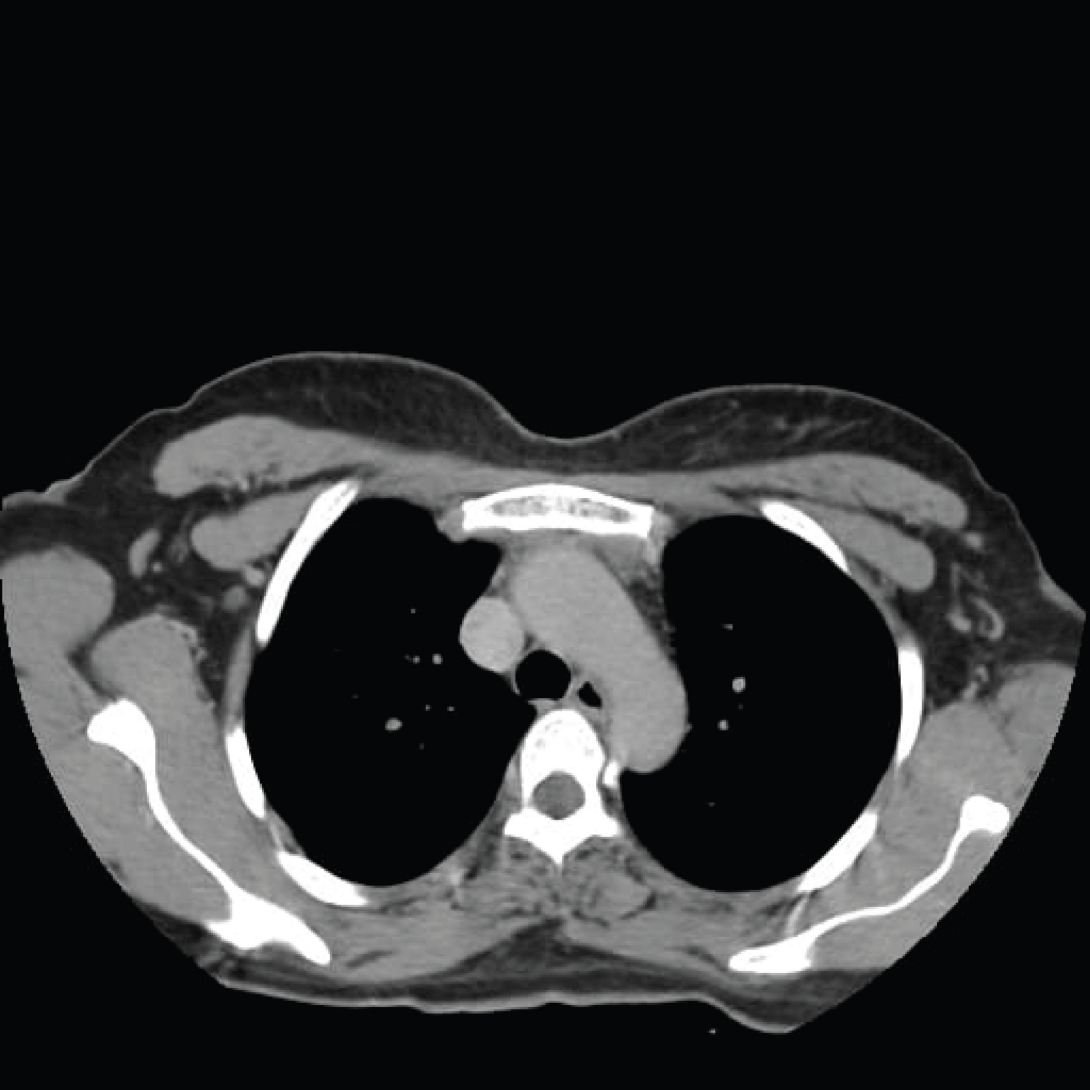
Follow-up axial thoracic CT scan showing complete resolution of the previously observed anterior mediastinal collection
